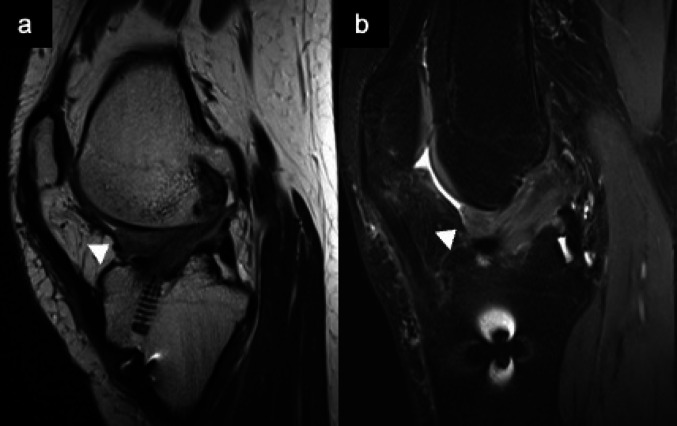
Cyclops lesion (Teenage female operated the ACL before 10 months). Sagittal T2-weighted (a) and fat suppressed T2-weighted (b) MR images demonstrate an intermediate signal intensity mass (arrowheads) located anterior to the intercondylar notch, near the tibial insertion of the cruciate ligaments
